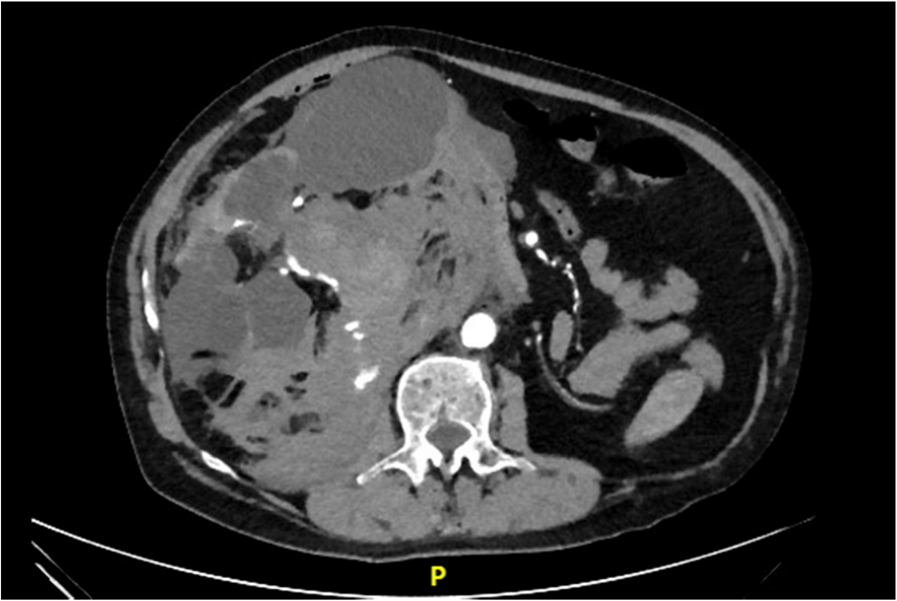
Axial CT scan of the abdomen and pelvis showing active contrast extravasation along the posterior aspect of a right perinephric hematoma extending into its most dependent aspect on the arterial phase associated with contrast pooling- consistent with an acute bleed; most likely arising from a right lower segment renal artery
